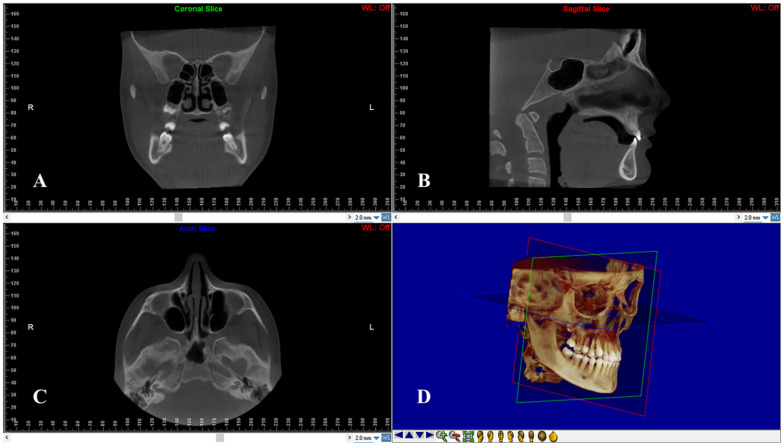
Head re-orientation on coronal (A), sagittal (B) and axial (C) planes of CBCT scans. The 3D image (D) shows the head orientation on a 3D space.
